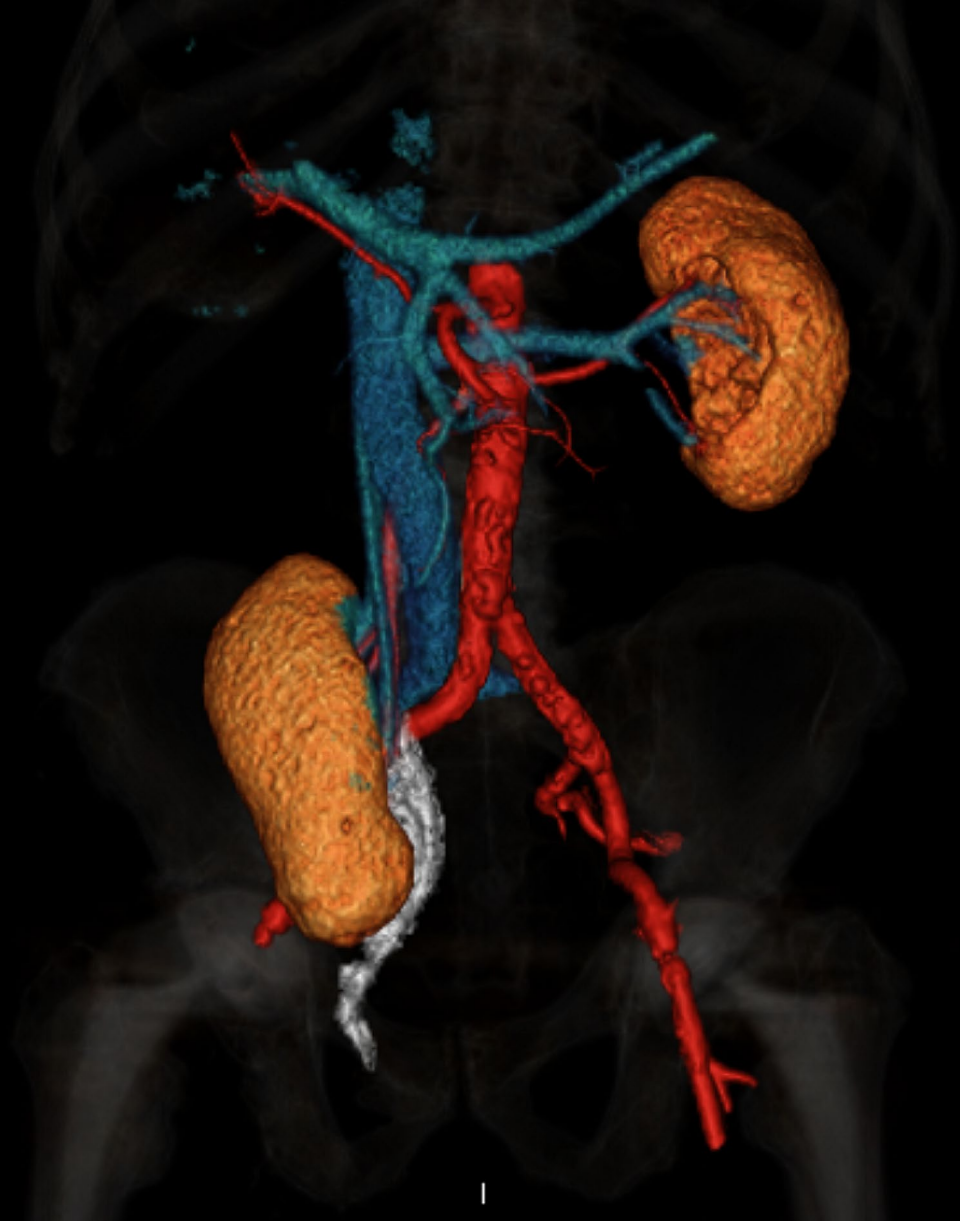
3D reconstruction of CT scan, showing ptotic right kidney with normal origin of renal vessels and ureter obstructed in inguinal ring
